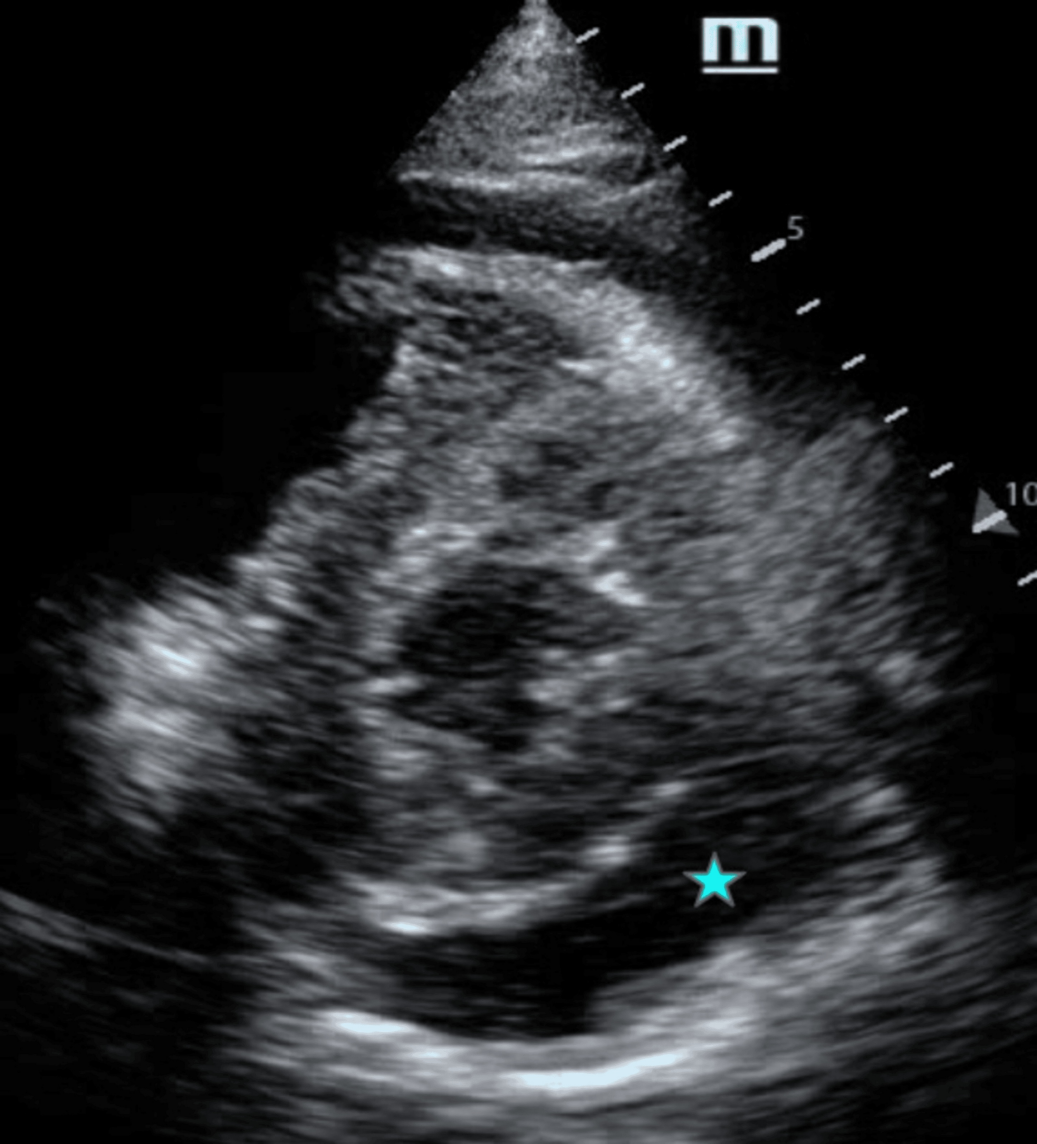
Parasternal short-axis view demonstrating a circumferential pericardial effusion (maximum diameter, 2 cm) Transthoracic echocardiography in the parasternal short-axis view demonstrating a circumferential pericardial effusion (blue asterisk) with an estimated maximum separation of approximately 2 cm between the pericardial layers.
